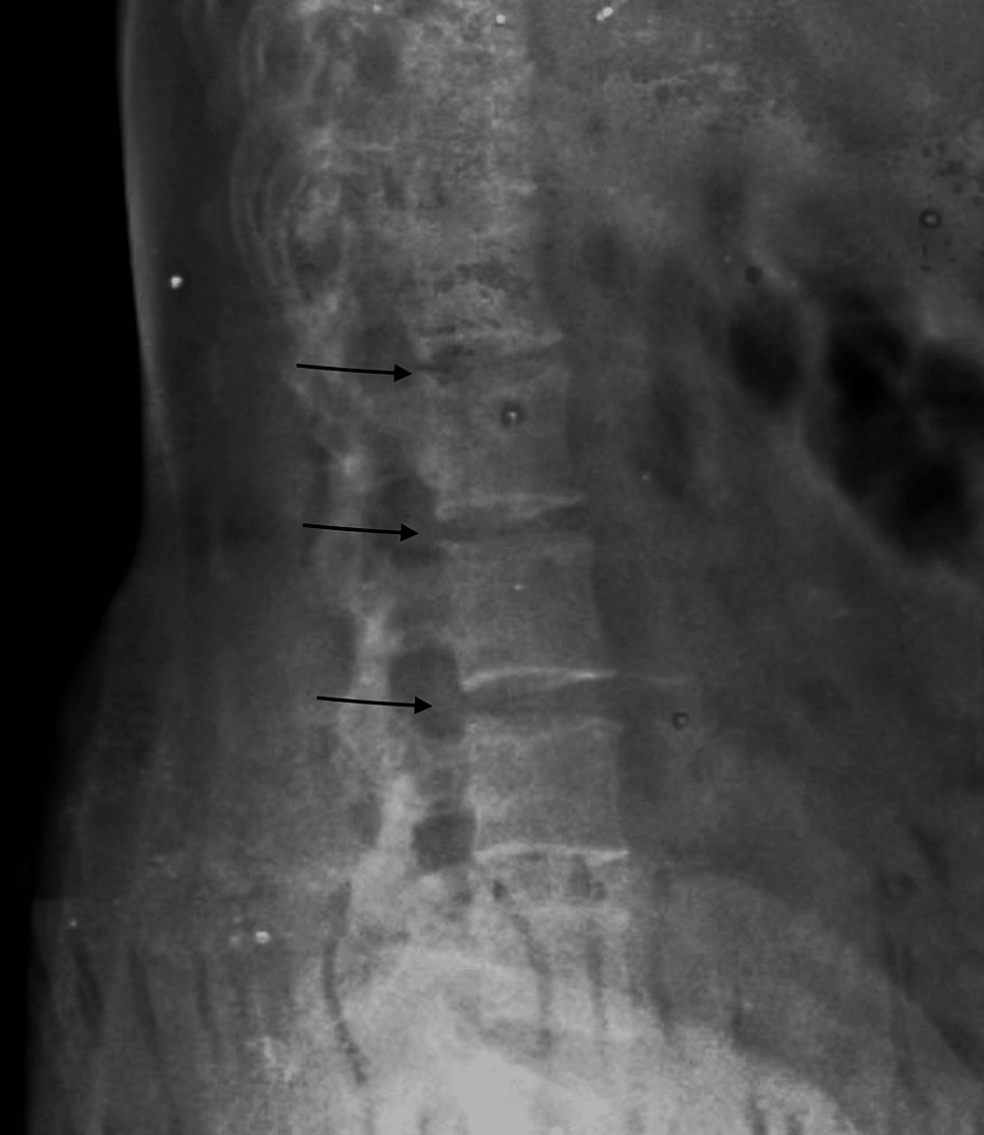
Lateral x-ray of the Lumbar Spine showing decreased intervertebral space
